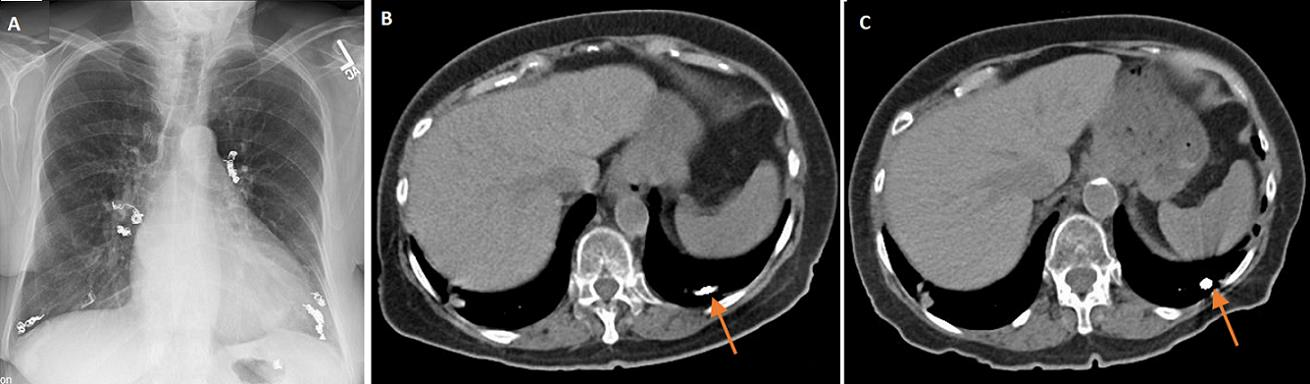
A) chest X-ray showing multiple embolization coils bilaterally; B) CT chest without contrast showing large AVM at left lower lobe (arrow); C) a repeat CT chest without contrast one year later showing smaller AVM at left lower lobe after coiling (arrow)
